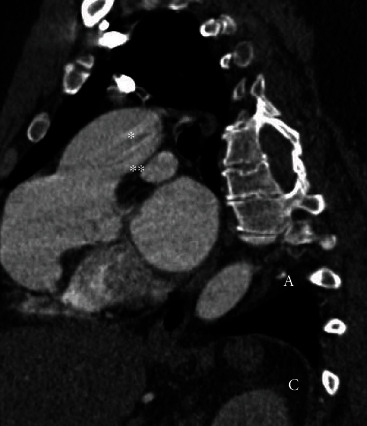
Transverse computed tomography angiography view of the aneurysm with a 2-dimensional diameter of 4.7 cm at the level of sinotubular junction.
